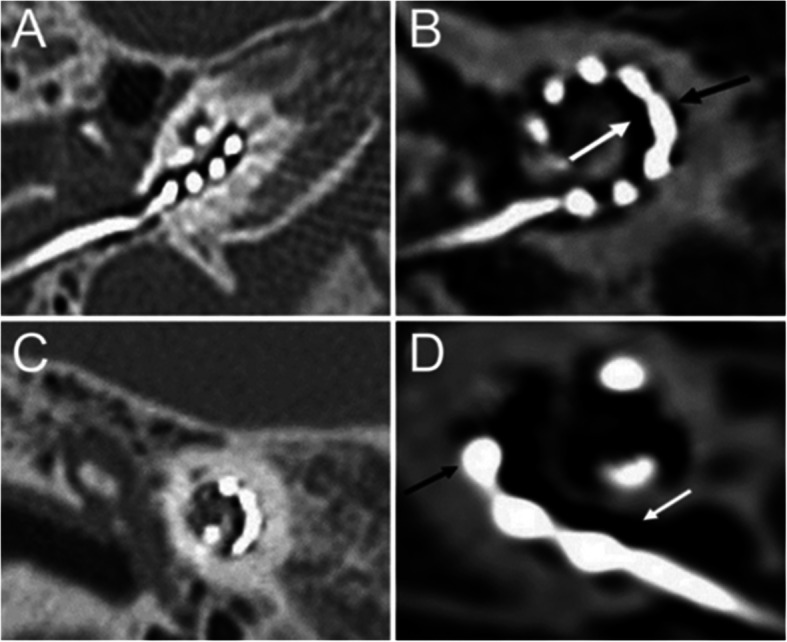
CT image of cochlea after using SEMAR combined with MPR. In the SEMAR CT image, the electrode is clearly located in the cochlea, it is difficult to evaluate whether the electrode is located in the scala tympani (a, c). Combining SEMAR with MPR enables clarification that the electrode is close to the cochlear wall. However, there is a clear spatial distance between the axes, thus the electrode is determined to be located in the scala tympani (b, d). The arrows indicate the electrode position
